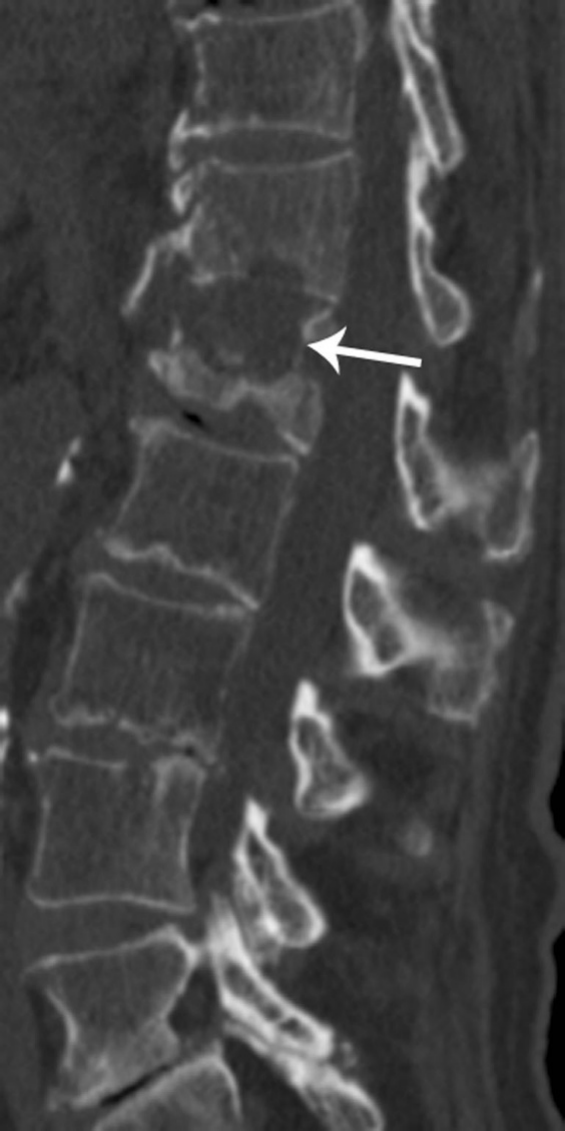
Lumbar CT-scan with sagittal reconstruction and bone windowing shows destruction of T12/L1 disk and erosion of both endplates (*white arrow*)
